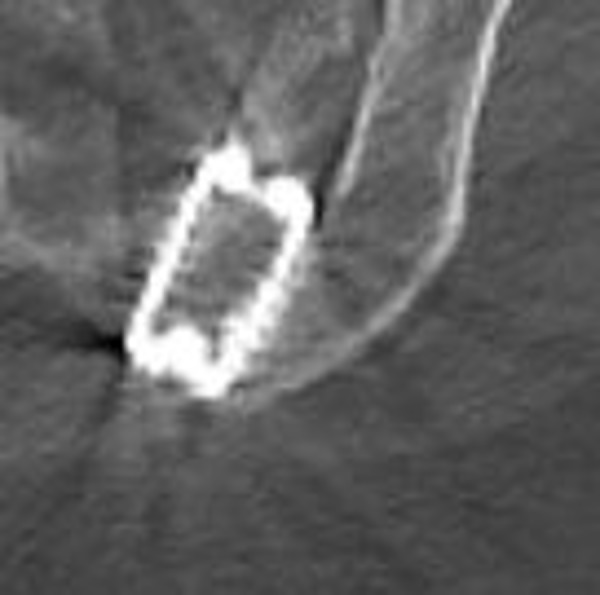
Axial CT of the left-sided posterior medial oblique fusion
